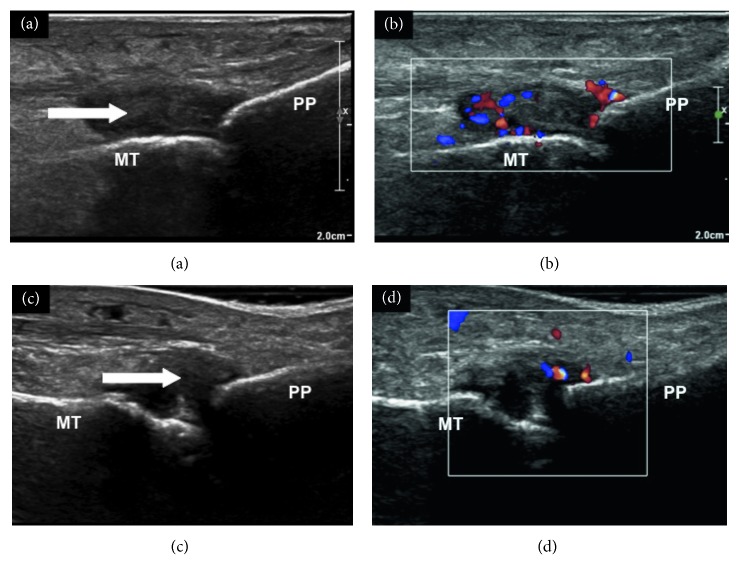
Musculoskeletal ultrasound images showing synovitis of right second and third metatarsophalangeal (MTP) joints. (a) Synovial hypertrophy of right second MTP joint (arrow); (b) increased Doppler signal indicating active inflammation; (c) synovial hypertrophy of right third MTP joint (arrow); (d) increased Doppler signal indicating active inflammation. MT: metatarsal; PP: proximal phalange.
